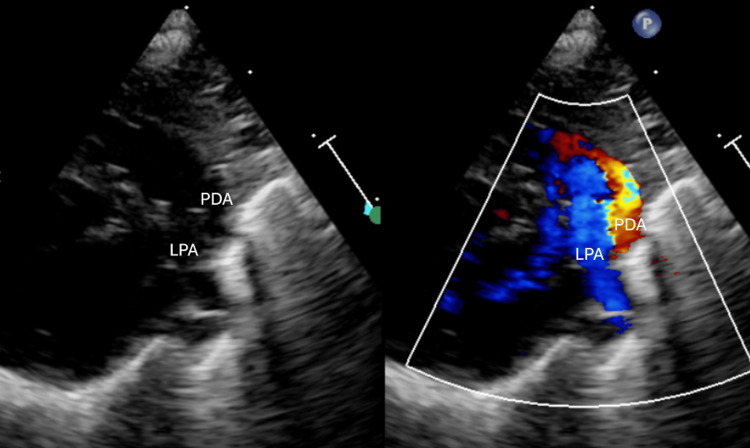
Echocardiography showed Type F PDA which characterized by a long, tortuous, and tubular morphology. Notably, it exhibits a distinct caudal angulation at the pulmonary artery insertion point.
